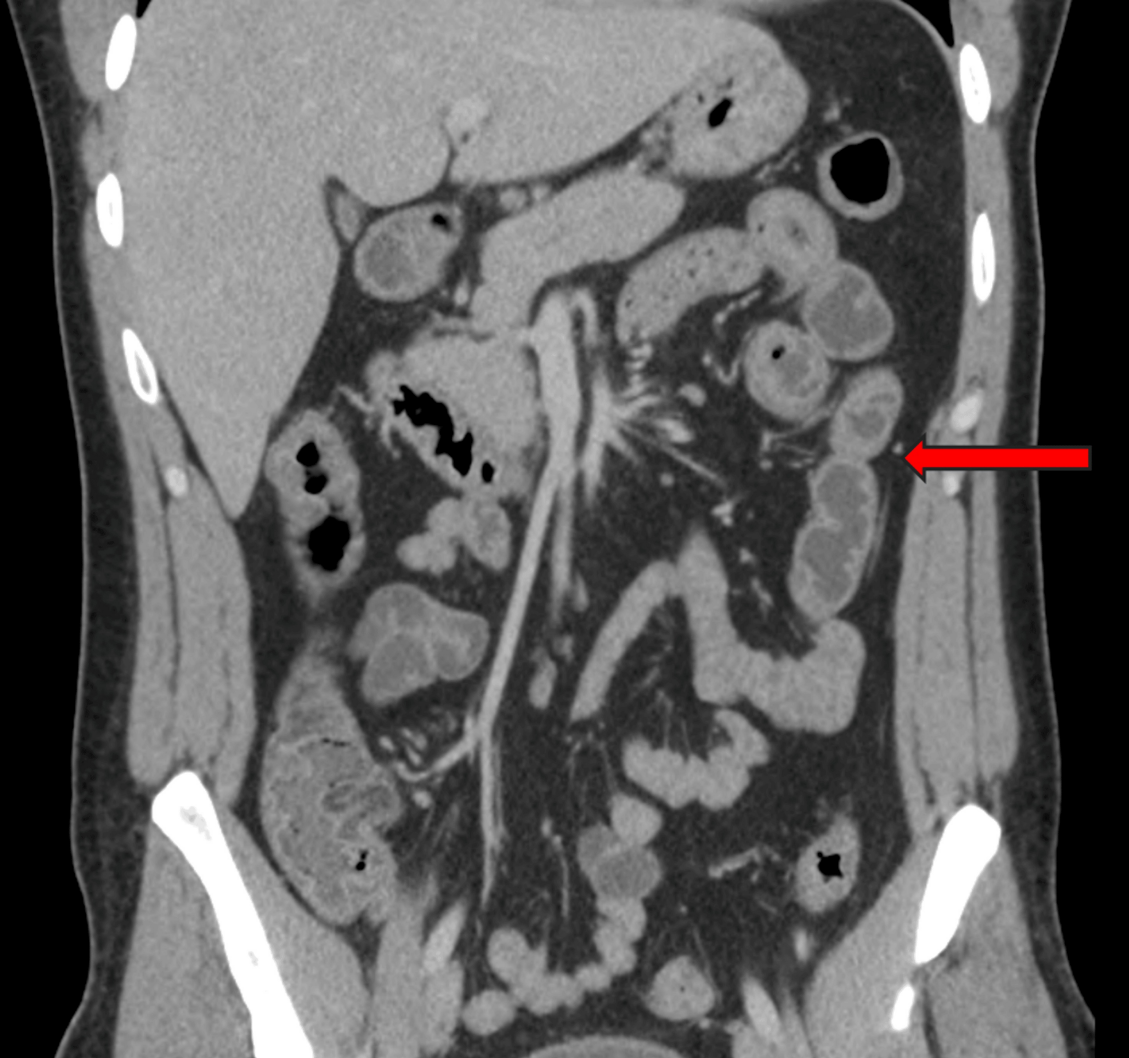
CT scan of the chest, abdomen, and pelvis with inflammatory changes in a section of the jejunum, as well as in the terminal ileum and colon The red arrow points towards an area of mucosal enhancement with increased wall thickness.
